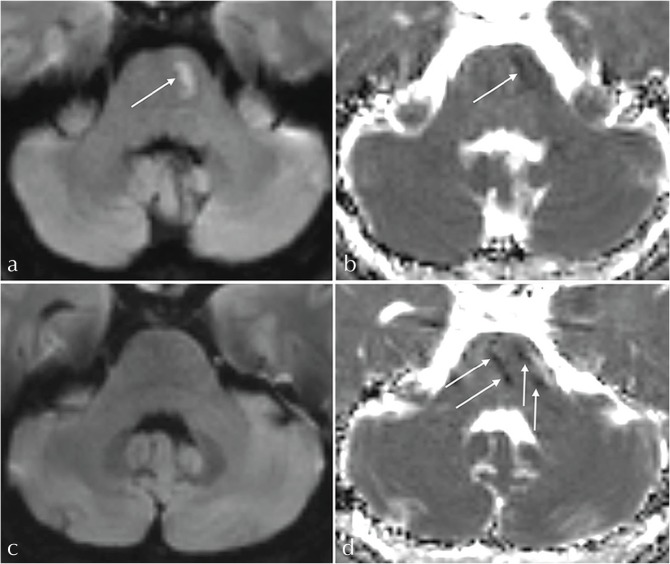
Examples of a true positive (a, b) and a false positive case (c, d). (a) Subacute infarction shows a high signal intensity (arrow) in the left side of the pons on the diffusion-weighted image (DWI) by readout-segmented EPI (rs-EPI) (b = 1000, number of readout segments [NRS] = 5). (b) On the apparent diffusion coefficient (ADC) map, this infarction shows slightly decreased values (arrow). (c) In another patient, there is no abnormal signal in the pons on the DWI obtained with the same parameters as (a). (d) Cerebrospinal fluid (CSF) pulsation artifacts are seen on the ADC map (arrows).
